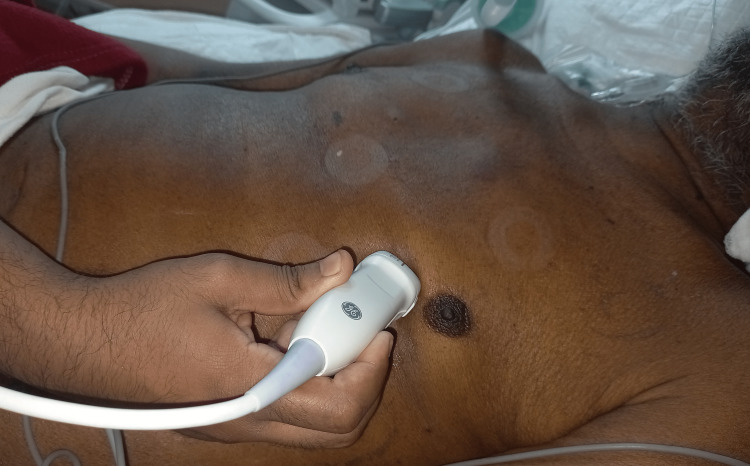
Probe position for apical five-chamber view (lateral view)
